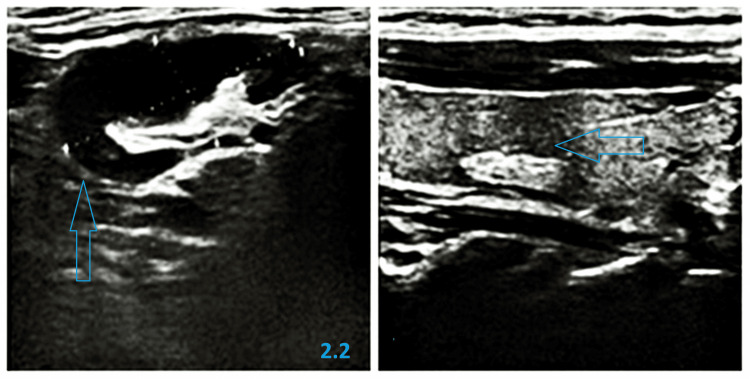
Ultrasound examination of enlarged left cervical lymph nodes 2.2 revealed thickened, hypoechoic cortex up to 36 mm in diameter Both sides: enlarged left cervical lymph nodes with thickened, hypoechoic cortex Left side (2.2): a lymph node with a diameter of 36 mm was observed
